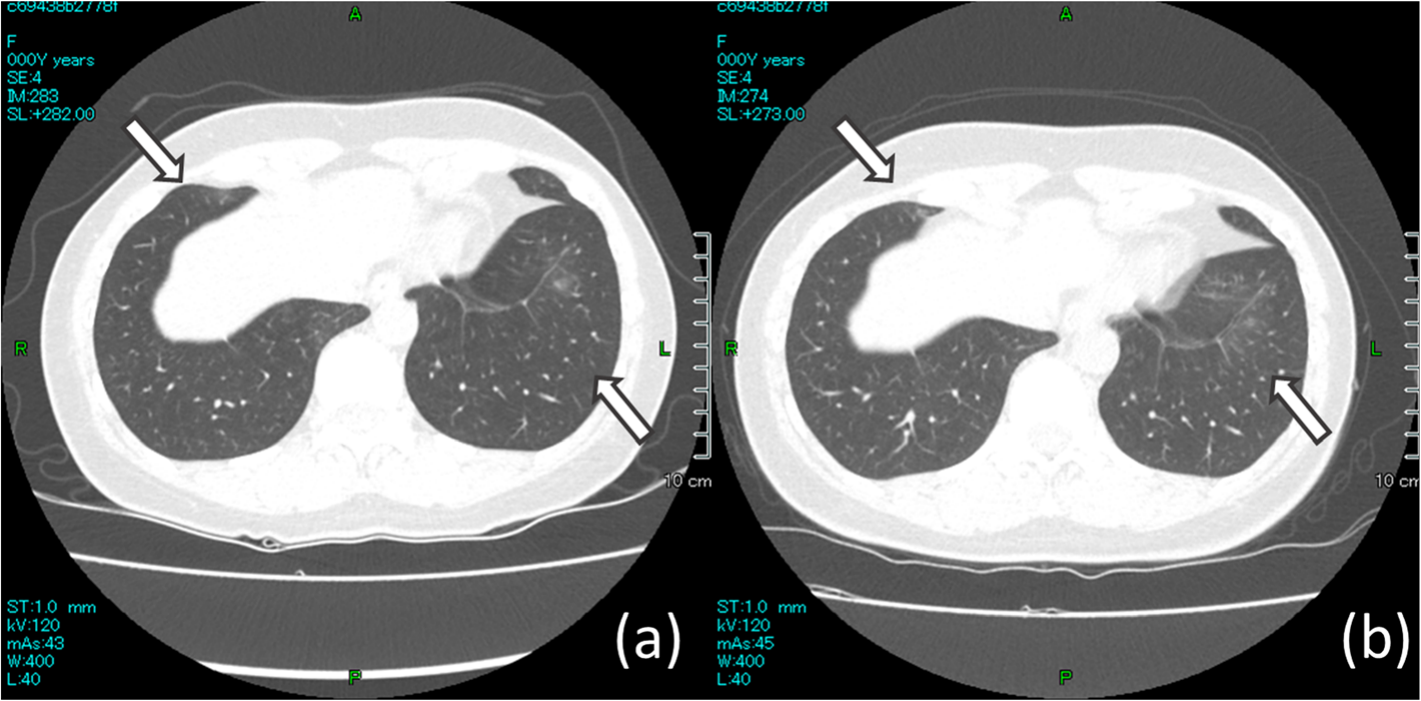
Computed tomography (CT) images of the lung field of Case 3. At the time of initial presentation (a) and 3 weeks later (b). CT revealed ground-glass opacity in the bilateral inferior lung fields (arrows) but no change over time
